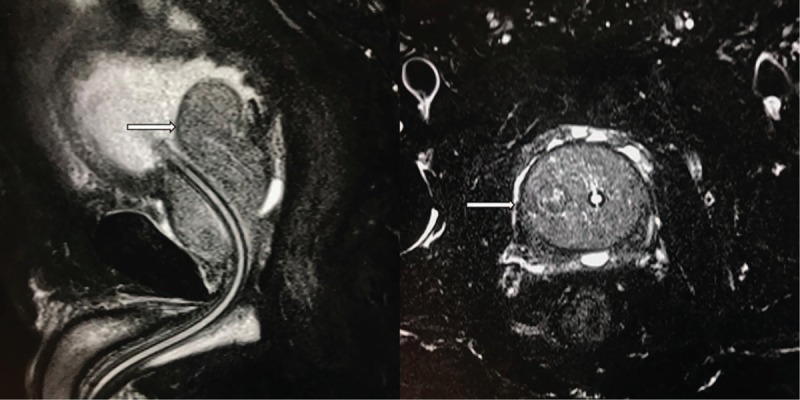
The MRI scan of the prostate showed the enlarged prostate gland (arrow) in T2 enhanced-weighted imaging.
